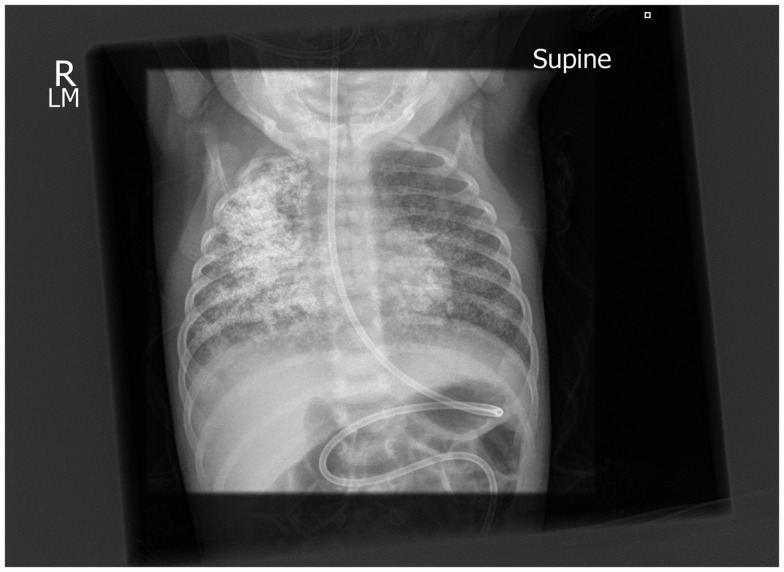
Post-aspiration chest x-ray showing well-defined radio-dense opacities through all zones of the right lung and similar but less extensive changes on the right.
